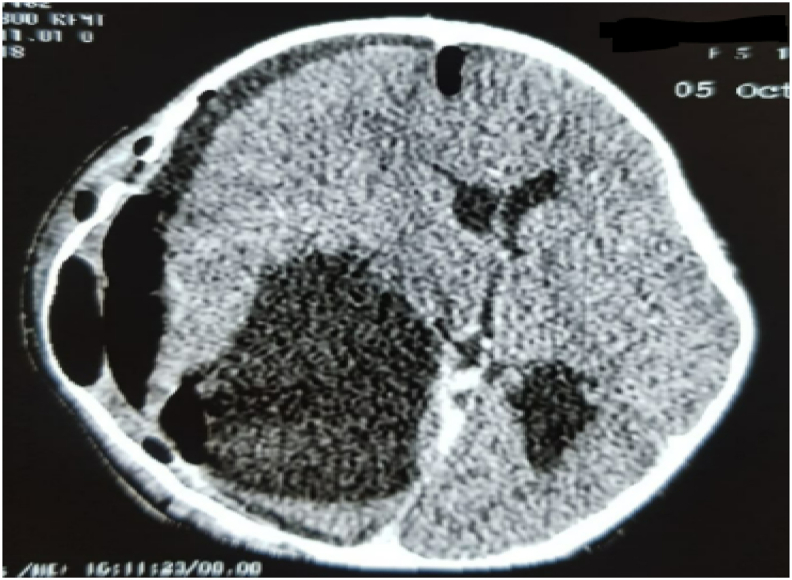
The control CT scan showing the total removal of the hydatid cyst.
